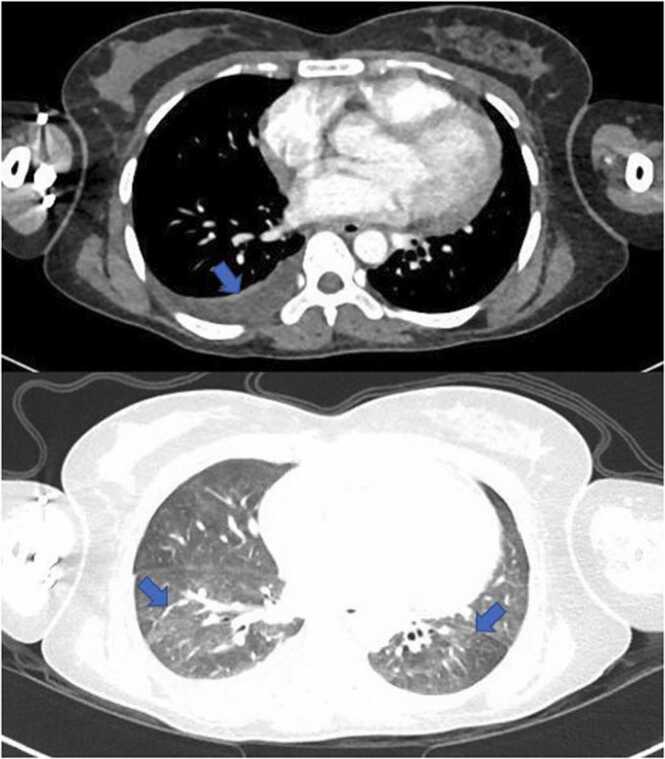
CT thorax showing right sided pleural effusion ( top), and bilateral ground-glass opacities mainly in the lower lung lobes(bottom).
